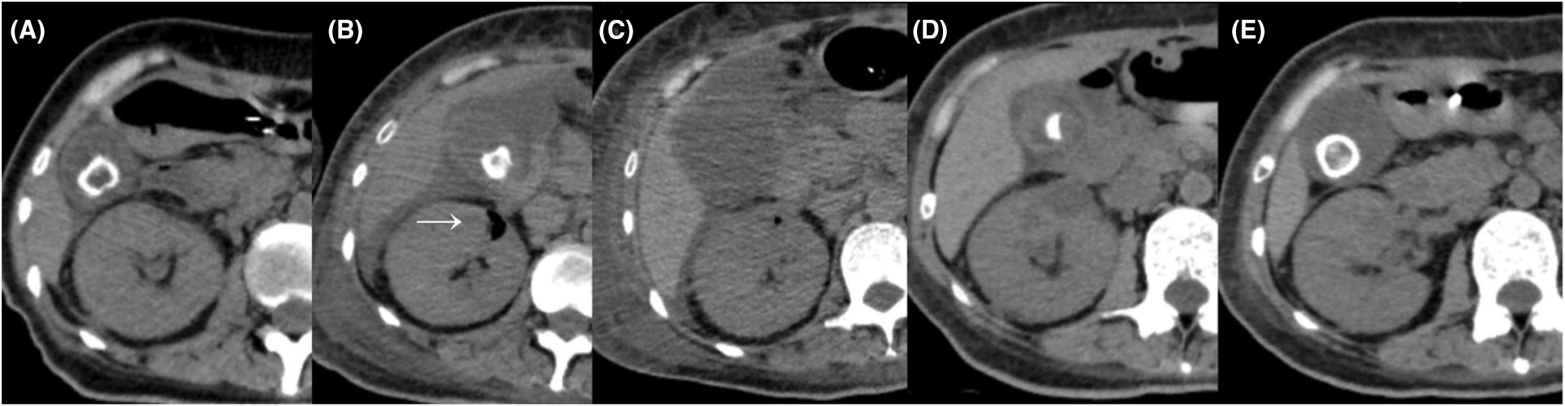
Progressive changes observed in computed tomography (CT) scan results over 49 days of hospitalization. Progressive changes were identified in CT radiographs on Day 0 (A), Day 3 (B), Day 10 (C), Day 18 (D), and Day 49 (E). The intrarenal gas detected on the 3rd day (B) (white arrow) disappeared on the 18th day (D).
